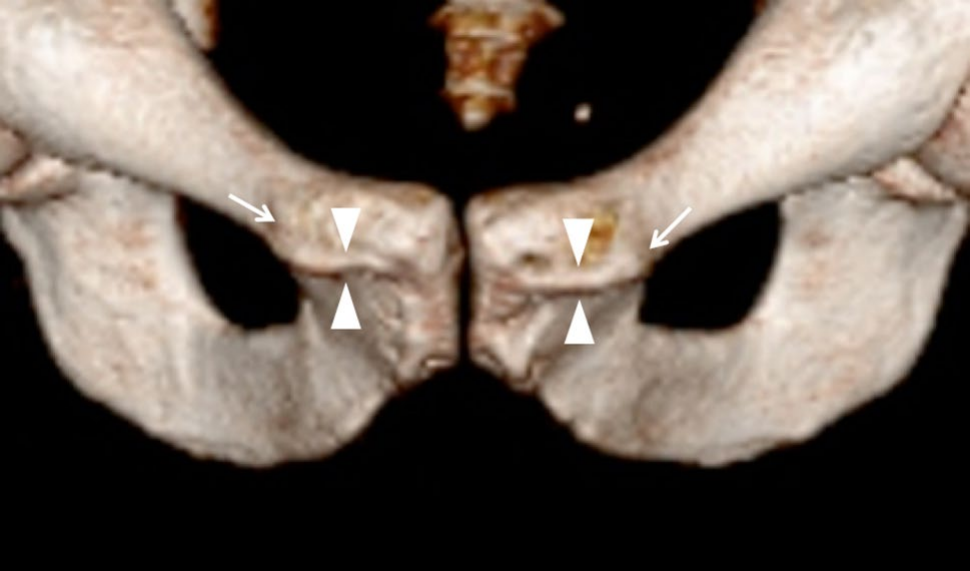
3D CT image of a male person showing the osteology of the anterior symphysis with the pubic crest (*arrowheads*) in between the pubic tubercles (*arrows*)
